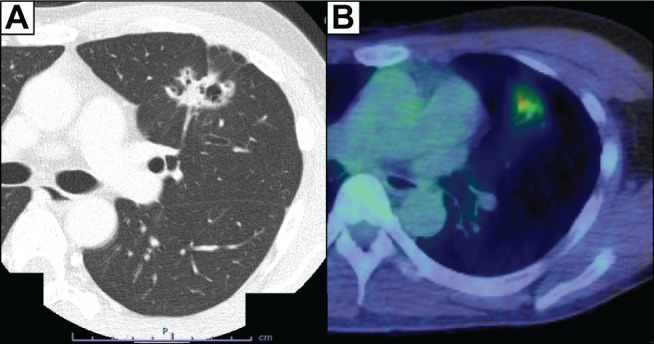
Preoperative CT. Contrast CT showed the pure-solid nodule (diameter: 3.3 cm) in the left lingular segment (A), and PET-CT showed fluorodeoxyglucose uptake, with an SUV max of 3.7 for the same nodule (B). PET, positron emission tomography
